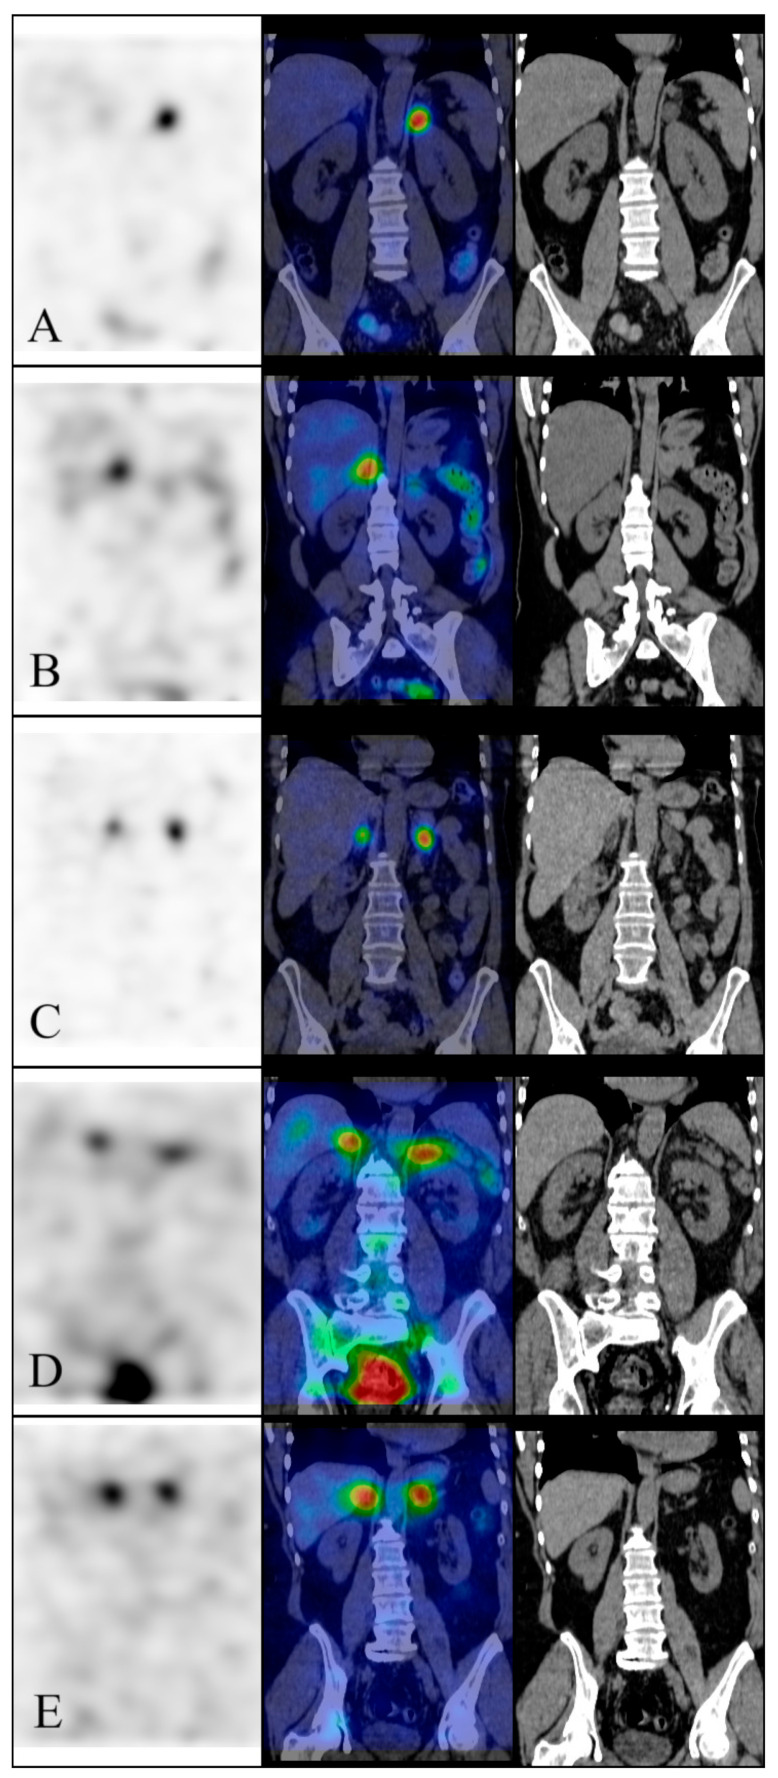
SPECT/CT images. (A): Unequivocal one-sided mineralocorticoid overproduction; (B–D): Cases with some indistinctness, where lateralization index calculation really helps; (E): Unequivocal two-sided mineralocorticoid overproduction. Lateralization indices: (A) 3.44, (B) 2.17, (C) 1.21, (D) 1.17, (E) 1.01. For each patient, SPECT (left), SPECT/CT fusion (middle) and CT (right) images are shown. Please see text for details of the technique.
